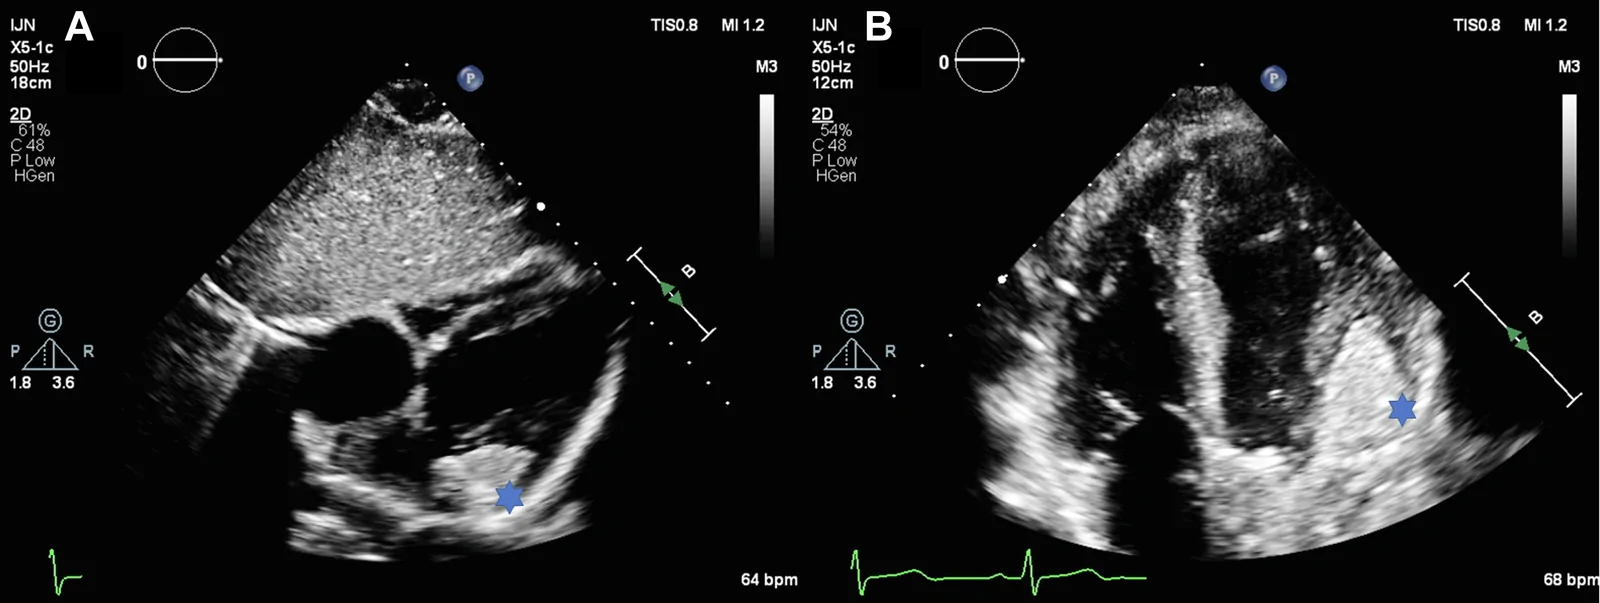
2D Postoperative Transthoracic Echocardiography (TTE) (A) Postoperative transthoracic echocardiogram subcostal view showing residual mass at the left ventricle. (B) Apical four-chamber view showing residual mass. Blue star refers to the residual mass seen on 2D postoperative transthoracic echocardiography. bpm = beats/minute; IJN = internal jugular vein; MI = myocardial infarction; TIS = tissue imaging system.
